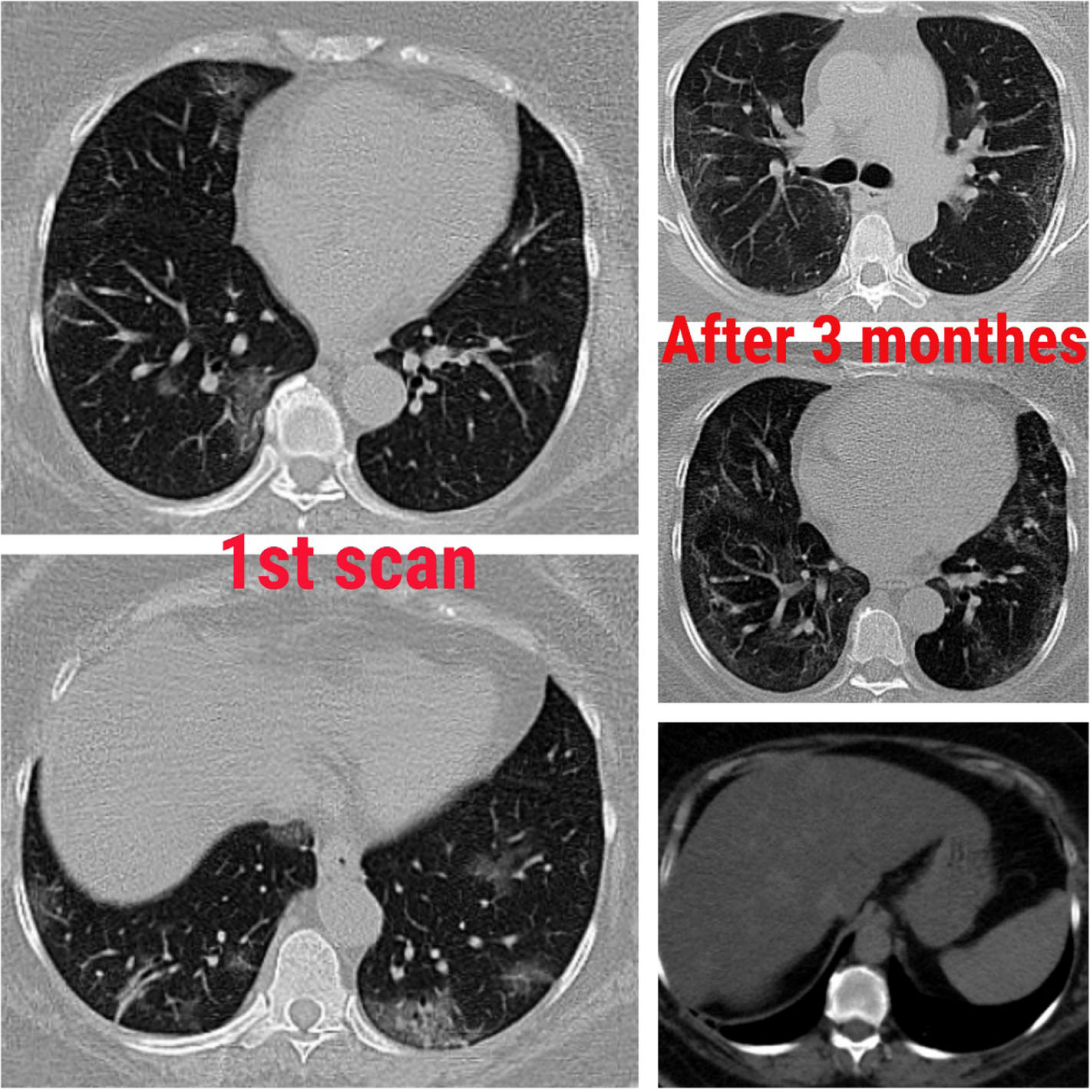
Female patient, 58 years old. The first scan shows multiple subpleural ground-glass opacities on follow-up after 3 months. CT shows multiple subpleural parenchymal bands. Upper abdominal cuts show fatty hepatomegaly
